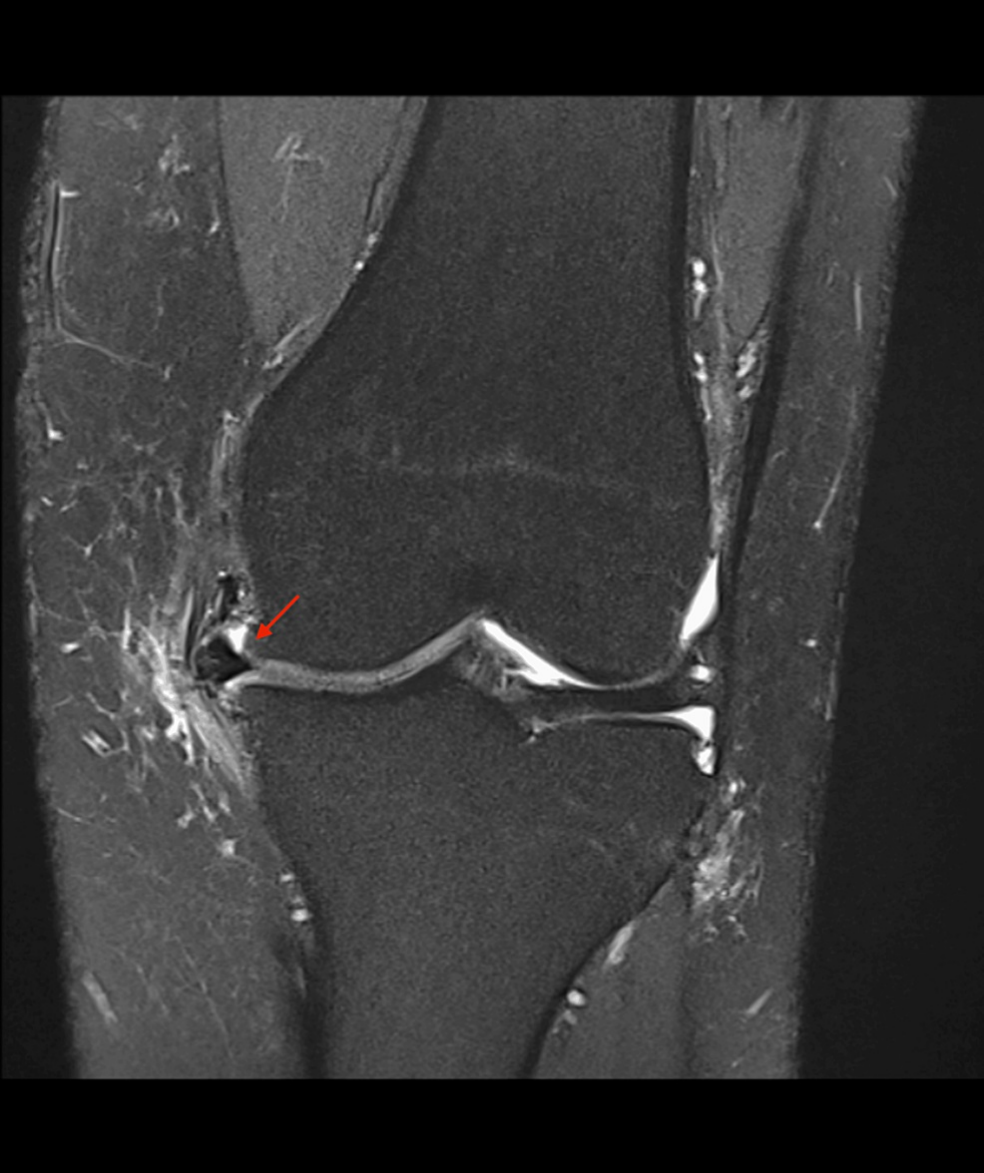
MRI three months post-MAT A 3 mm radial displacement (red arrow) of the meniscus corpus is visible. Coronal view of the left knee, medial meniscus. MAT: Meniscus allograft transplantation; MRI: magnetic resonance imaging
